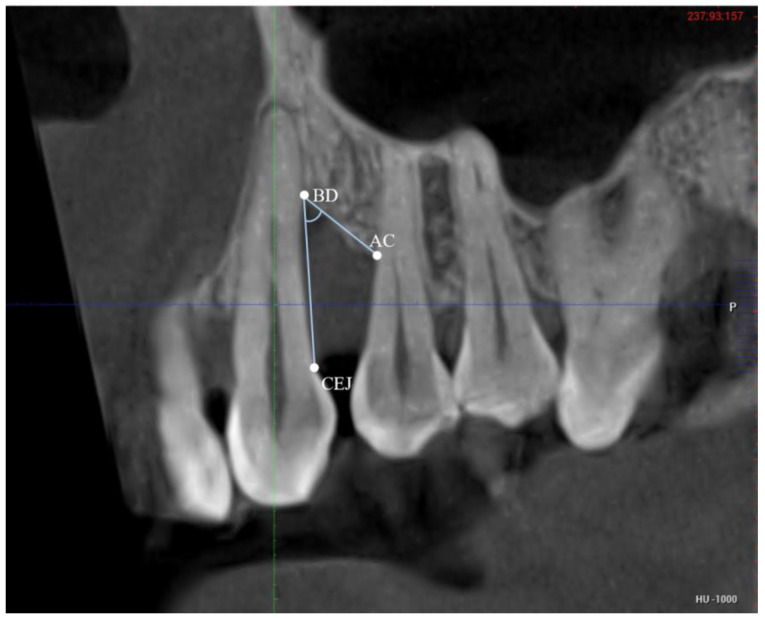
Example of a CBCT cut used for angle measurement. CEJ = cementoenamel junction; AC = most coronal point of adjacent bone crest; BD = base of defect. The defect angle is the angle between the lines CEJ-BD and AC-BD.
